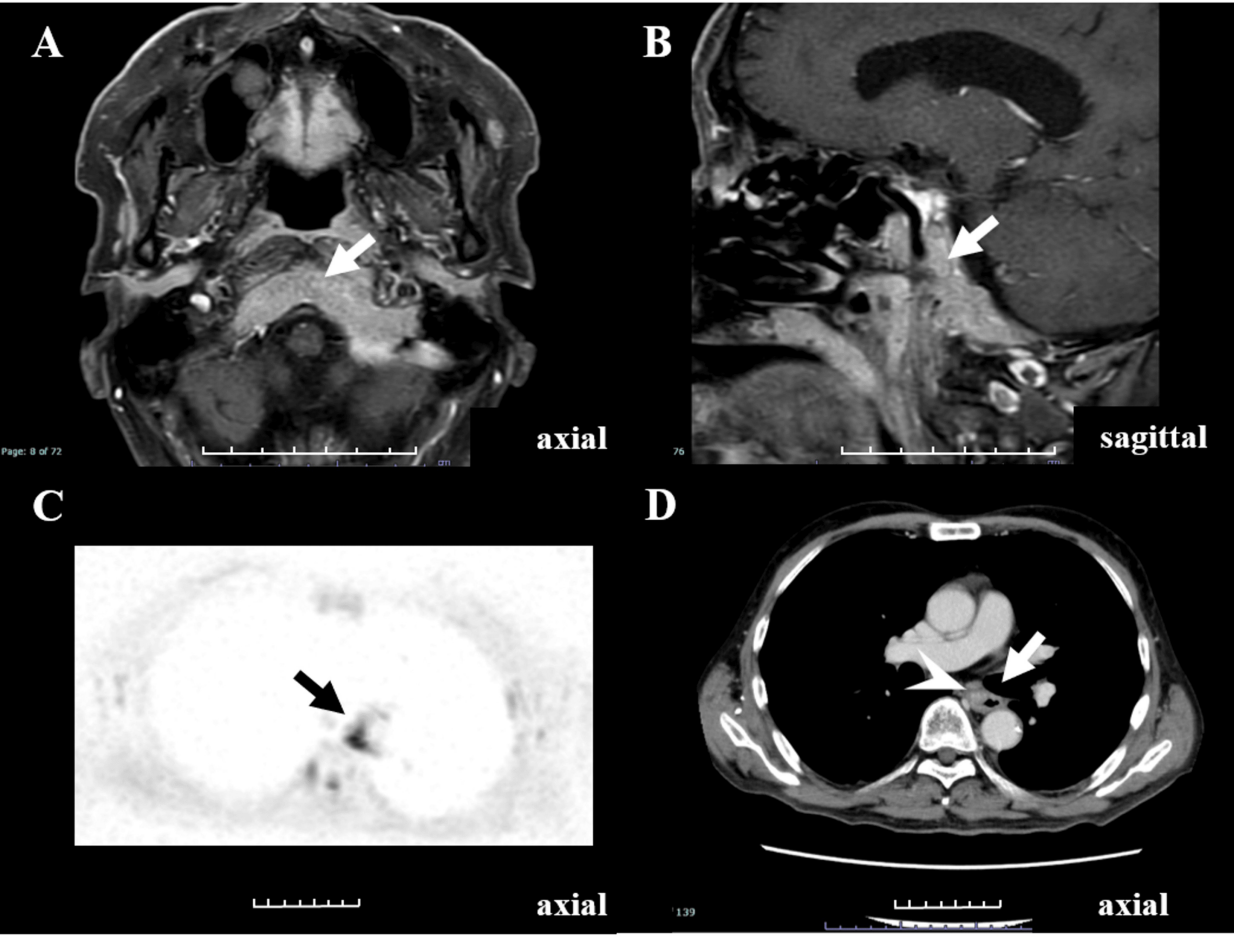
Brain MRI, DWIBS, chest contrast-enhanced CT images Initial (A) axial and (B) sagittal contrast-enhanced T1-weighted MRI. The clivus shows uniform enhancement, suggesting metastasis (white arrow); (C) Diffusion-weighted whole-body imaging with background body signal suppression (DWIBS) with b=800 s/mm² reveals high signal intensity (black on this image) in the esophagus (black arrow); contrast-enhanced chest computed tomography shows contrast enhancement in the same region, suggesting esophagus cancer (primary tumor); (D) White arrowhead indicates enhancing esophageal wall thickening with suspected adventitial extension. The lesion is indicated in each panel by an arrow. Each division on the scale bar is 1 cm.
